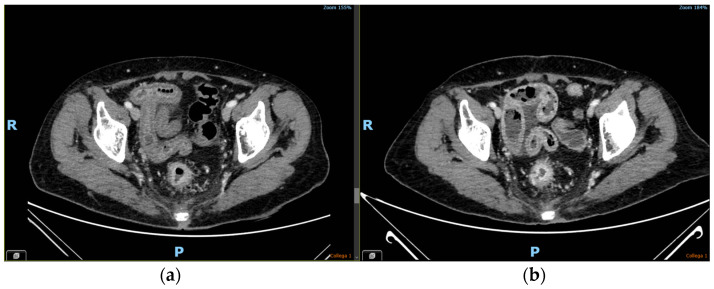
Abdominal computed tomography scans performed at the onset of the symptoms (a) and 12 days later (b); the former shows a massive ileal wall thickening with hyperemia of the mucosa and perivisceral vascular enhancement, findings that can still be observed (although in reduction) in the latter.
